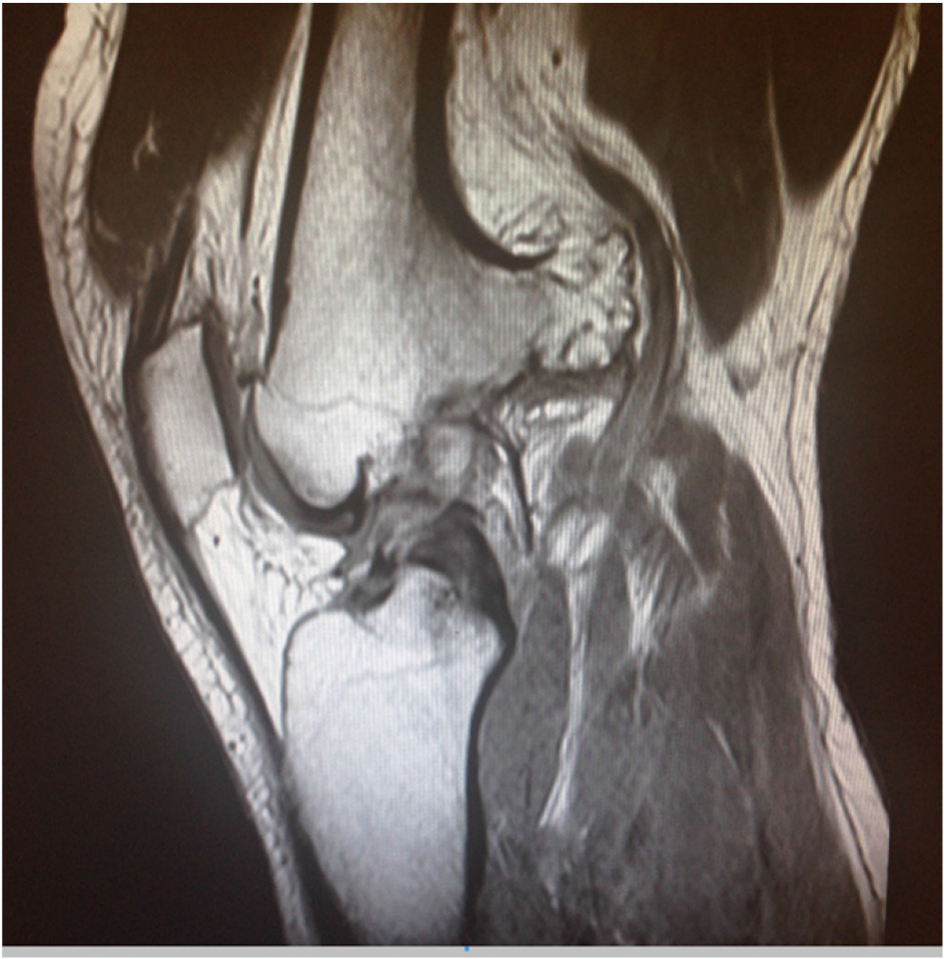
Sagittal MRI imaging of the left knee demonstrates the extent of the osteochondroma and the impact on surrounding structures.
